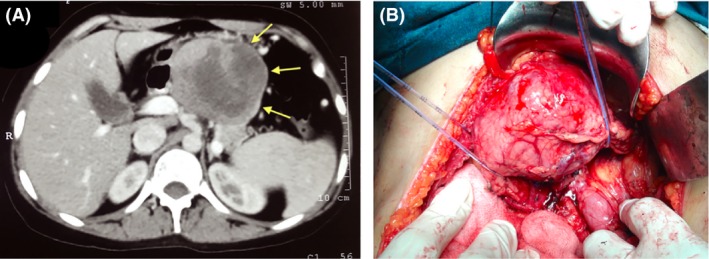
(A) Computed tomography showing a solid–cystic tumor arising from the body of pancreas. On exploration, a well‐circumscribed capsulated tumor was found arising from the body of pancreas (B).
